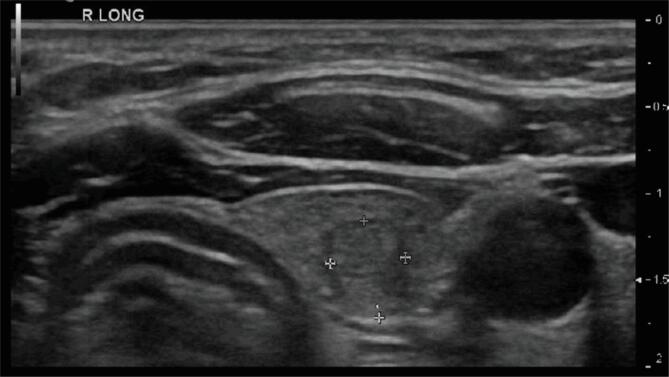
Thyroid Ultrasound showing an ill-defined, low attenuation 5mm nodule in the lower left lobe (single column width).
